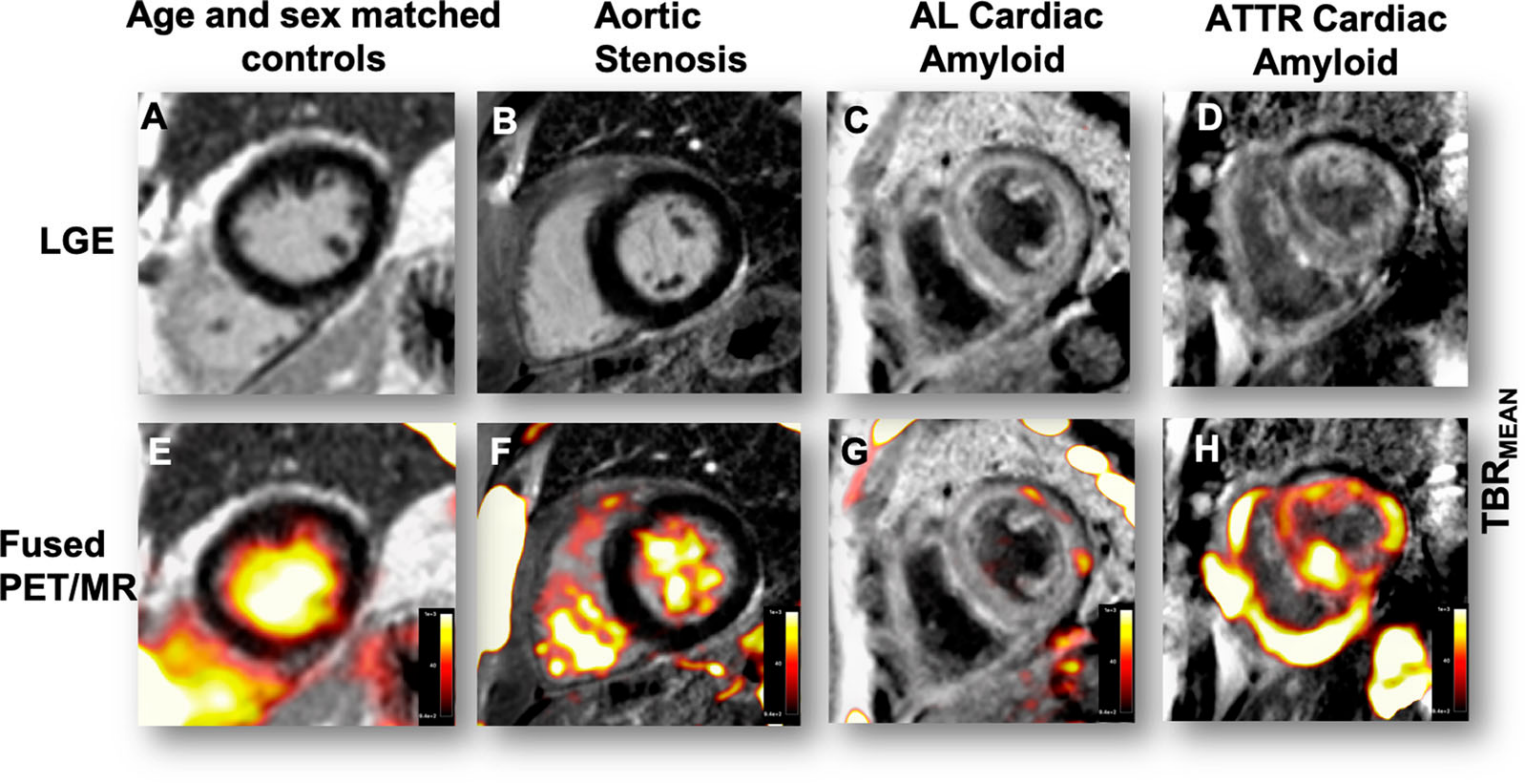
Patterns of 18F-fluoride uptake between cohorts. Columns represent each cohort and rows imaging modality in the short axis view. Panel A shows a delayed enhanced image of a control subject with normal myocardial mass and no LGE. The corresponding fused PET/MR image (E) shows uptake only in the blood pool. Panel B is a patient with aortic stenosis and elevated LV mass. Note the absence of myocardial 18F-fluoride uptake on panel F and similar to the healthy control, uptake is greater in the blood pool than myocardium. Panel C shows a patient with AL amyloid displaying the characteristic myocardial nulling difficulties with LGE found in cardiac amyloidosis. Panel G shows patchy lateral wall uptake greater than the blood pool. Panel D shows similar LGE findings, but this time in TTR amyloid. Note the striking and extensive biventricular uptake in panel H, much greater than the blood pool and what was seen in AL
